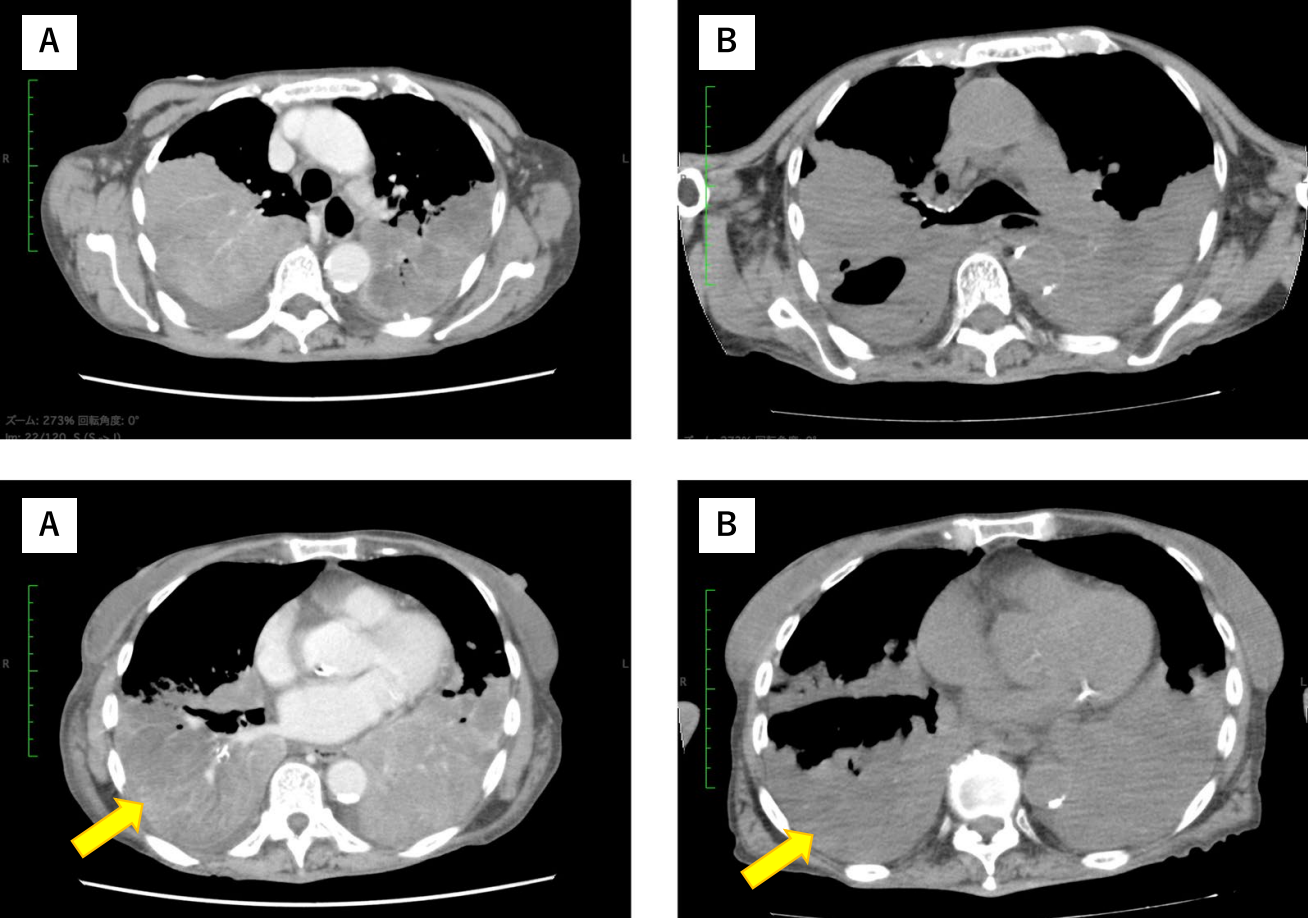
Chest computed tomography images before and after administering dabrafenib plus trametinib. The findings revealed a primary lung cancer and separate tumor nodules in the contralateral lobe before treatment and b slight tumor shrinkage in the right middle lobe (long arrow) without changes in the other lesions.
